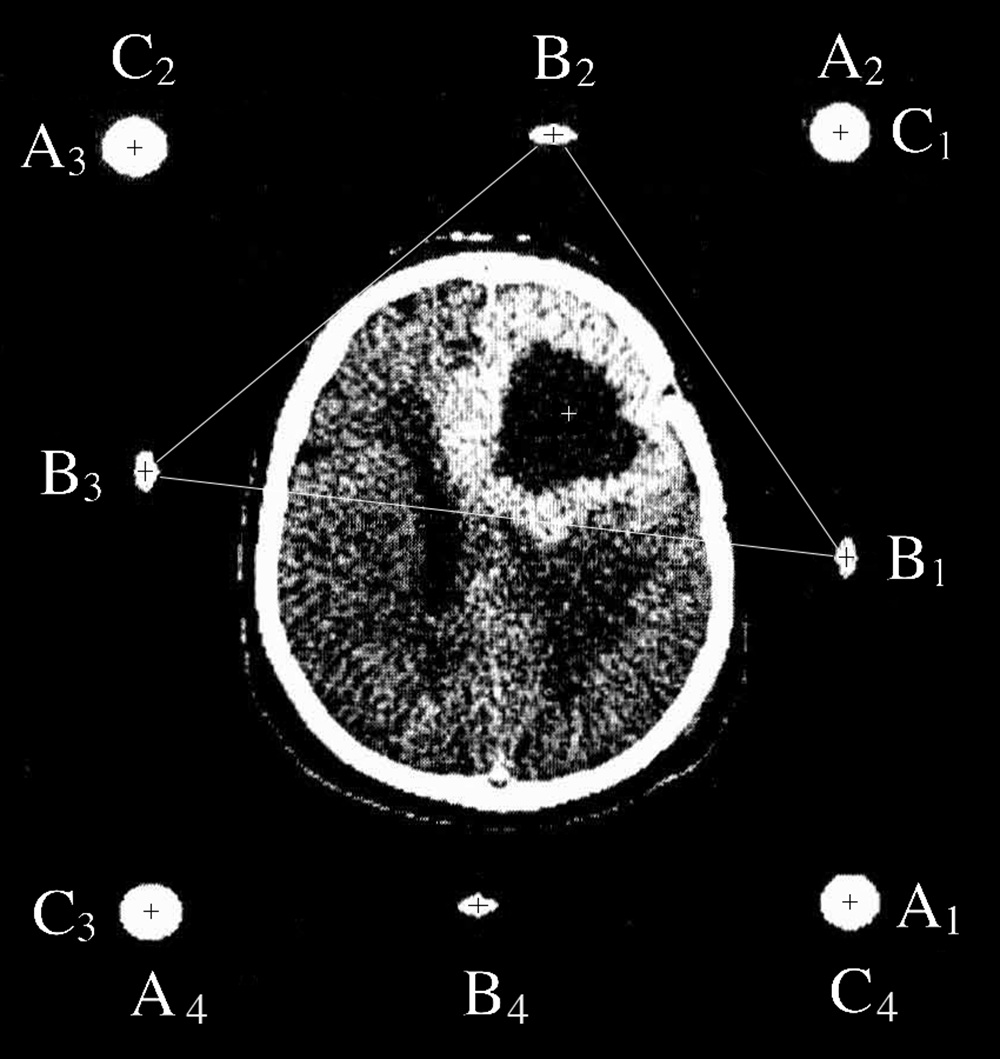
CT Image with Four Sets of Fiducials Four N-localizers create four sets of fiducials \begin{document}\left\{ \mathrm{A_1,B_1,C_1} \right\}\end{document}, \begin{document}\left\{ \mathrm{A_2,B_2,C_2} \right\}\end{document}, \begin{document}\left\{ \mathrm{A_3,B_3,C_3} \right\}\end{document}, and \begin{document}\left\{ \mathrm{A_4,B_4,C_4} \right\}\end{document}. The N-localizers are merged end-to-end such that \begin{document}\mathrm{A_1=C_4} \end{document}, \begin{document} \mathrm{A_2=C_1} \end{document}, \begin{document} \mathrm{A_3=C_2} \end{document}, and \begin{document}\mathrm{A_4=C_3} \end{document}. The black cross hairs indicate the centers of the fiducials and the white cross hairs indicate the target point \begin{document}P_T\end{document} that lies inside the triangle \begin{document} \mathrm{B_1B_2B_3} \end{document} (see text for explanation). Adapted from [9].
